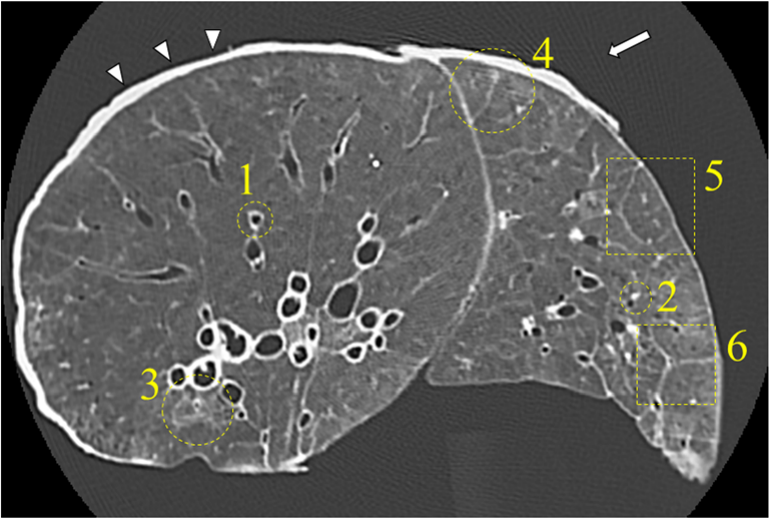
Evaluation items on reference images. On each reference image (U-HRCT_*N*_), each visual evaluation item to be scored is indicated using colored marks. This U-HRCT_*N*_ image of diffuse alveolar damage shows six evaluation items: 1, bronchi; 2, vessels; 3, ground-glass opacity; 4-6, interlobular septal thickening. Streak (arrow) and dark band artifacts (arrowhead) can also be seen. U-HRCT_*N*_: ultra-high-resolution CT with normal mode
